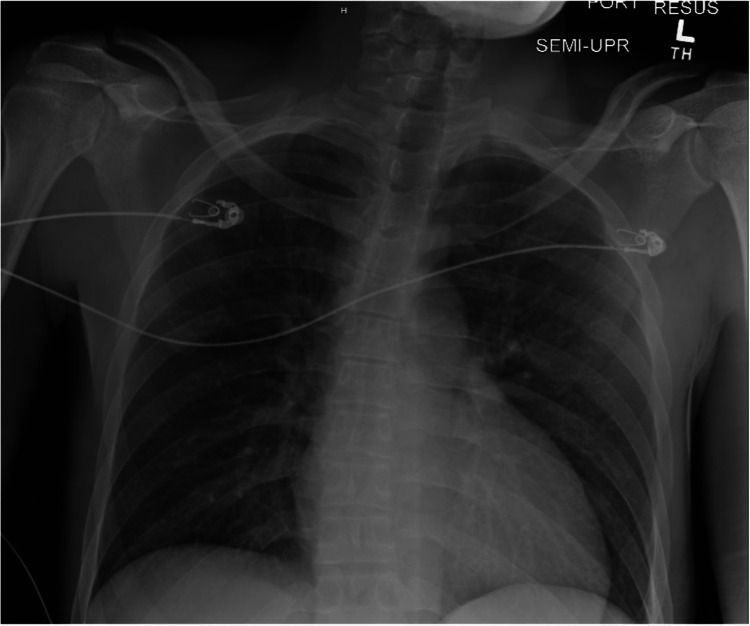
Image of the initial chest X-ray taken on admission There was no evidence of any acute consolidation or localization of bacterial infection that would indicate any pulmonary process from the initial read
